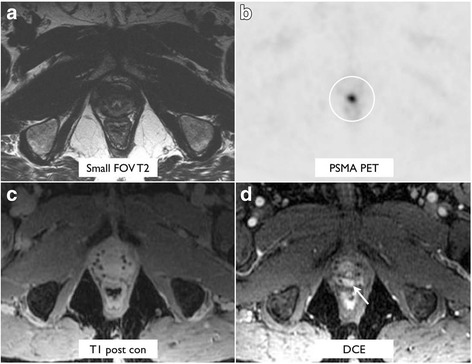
A 62-year-old man with biochemical recurrence (PSA of 3.2), who had suspected PSMA-avid prostate bed recurrence measuring 1.0 × 0.6 cm after initial treatment with brachytherapy, seen on PSMA PET (b) and DCE images (d). The local recurrence was not seen on T2-weighted imaging (a), post-gadolinium T1-weighted imaging (c), or DWI (not shown due to artifact from brachytherapy seeds)
